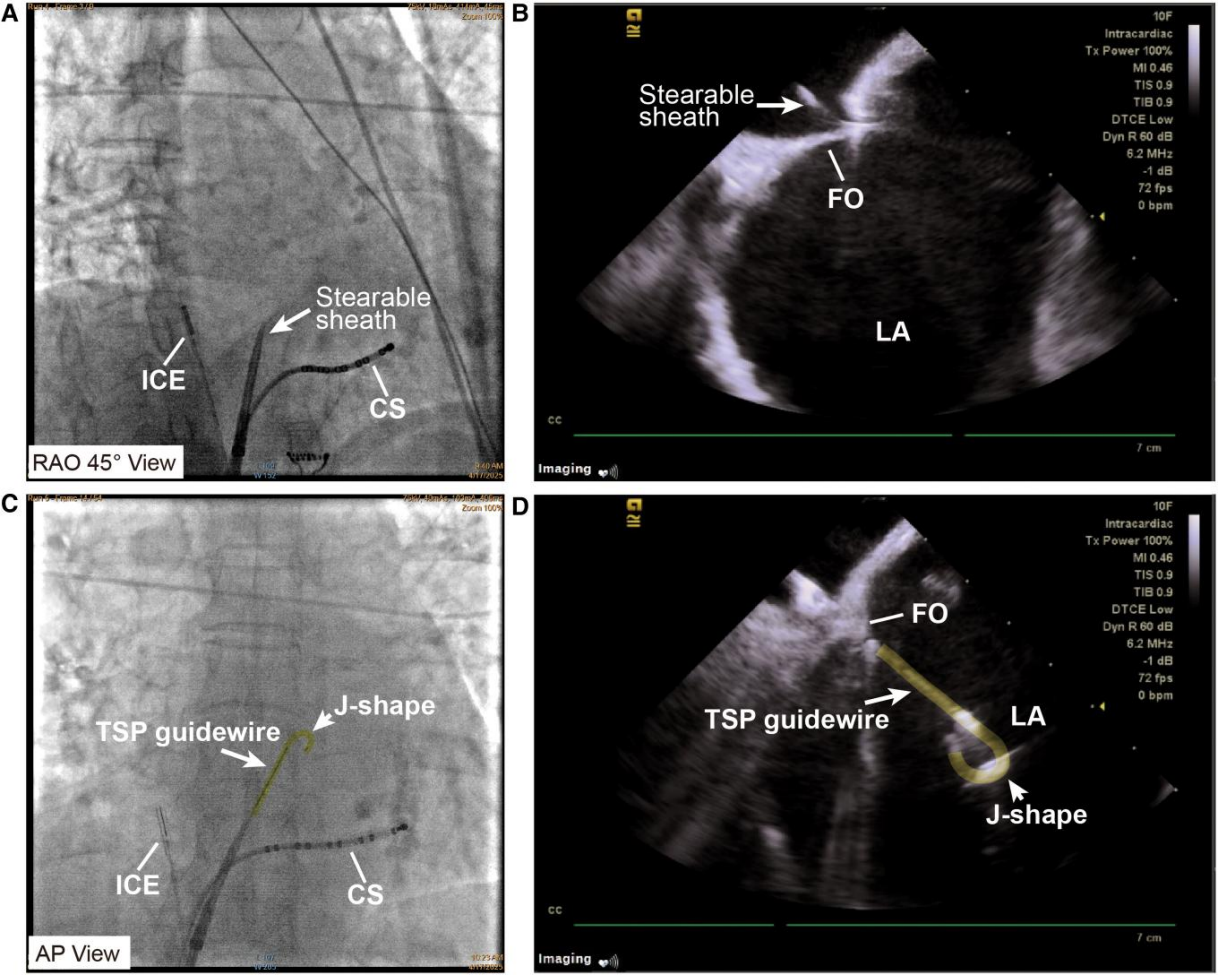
Successful transseptal puncture procedure with a novel transseptal wire. (*A* and *B*) A steerable sheath (CARTO VIZIGO™, Biosense Webster, USA) was advanced to the fossa ovalis under X-ray fluoroscopy (*A*, white arrow) and intracardiac echocardiography guidance (*B*, white arrow). (*C* and *D*) Attempts of transseptal puncture with a transseptal puncture guidewire (AccuSafe™, Synaptic Medical, yellow markings). A ‘J shape’ (short white arrows) formed in the distal part of wire (long white arrows) automatically after transseptal puncture, which was observed clearly under X-ray fluoroscopy (*C*) and intracardiac echocardiography (*D*). CS, coronary sinus catheter; FO, fossa ovalis; ICE, intracardiac echocardiography; LA, left atrium; TSP, transseptal puncture.
